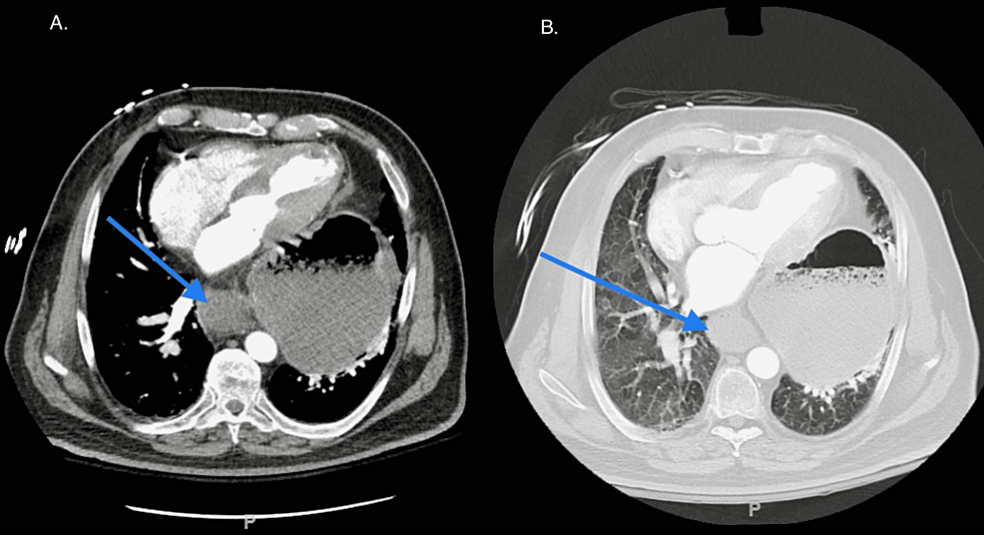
Transverse section of CT chest demonstrating a large hiatal hernia and a dilated, fluid-filled esophagus (blue arrows).
